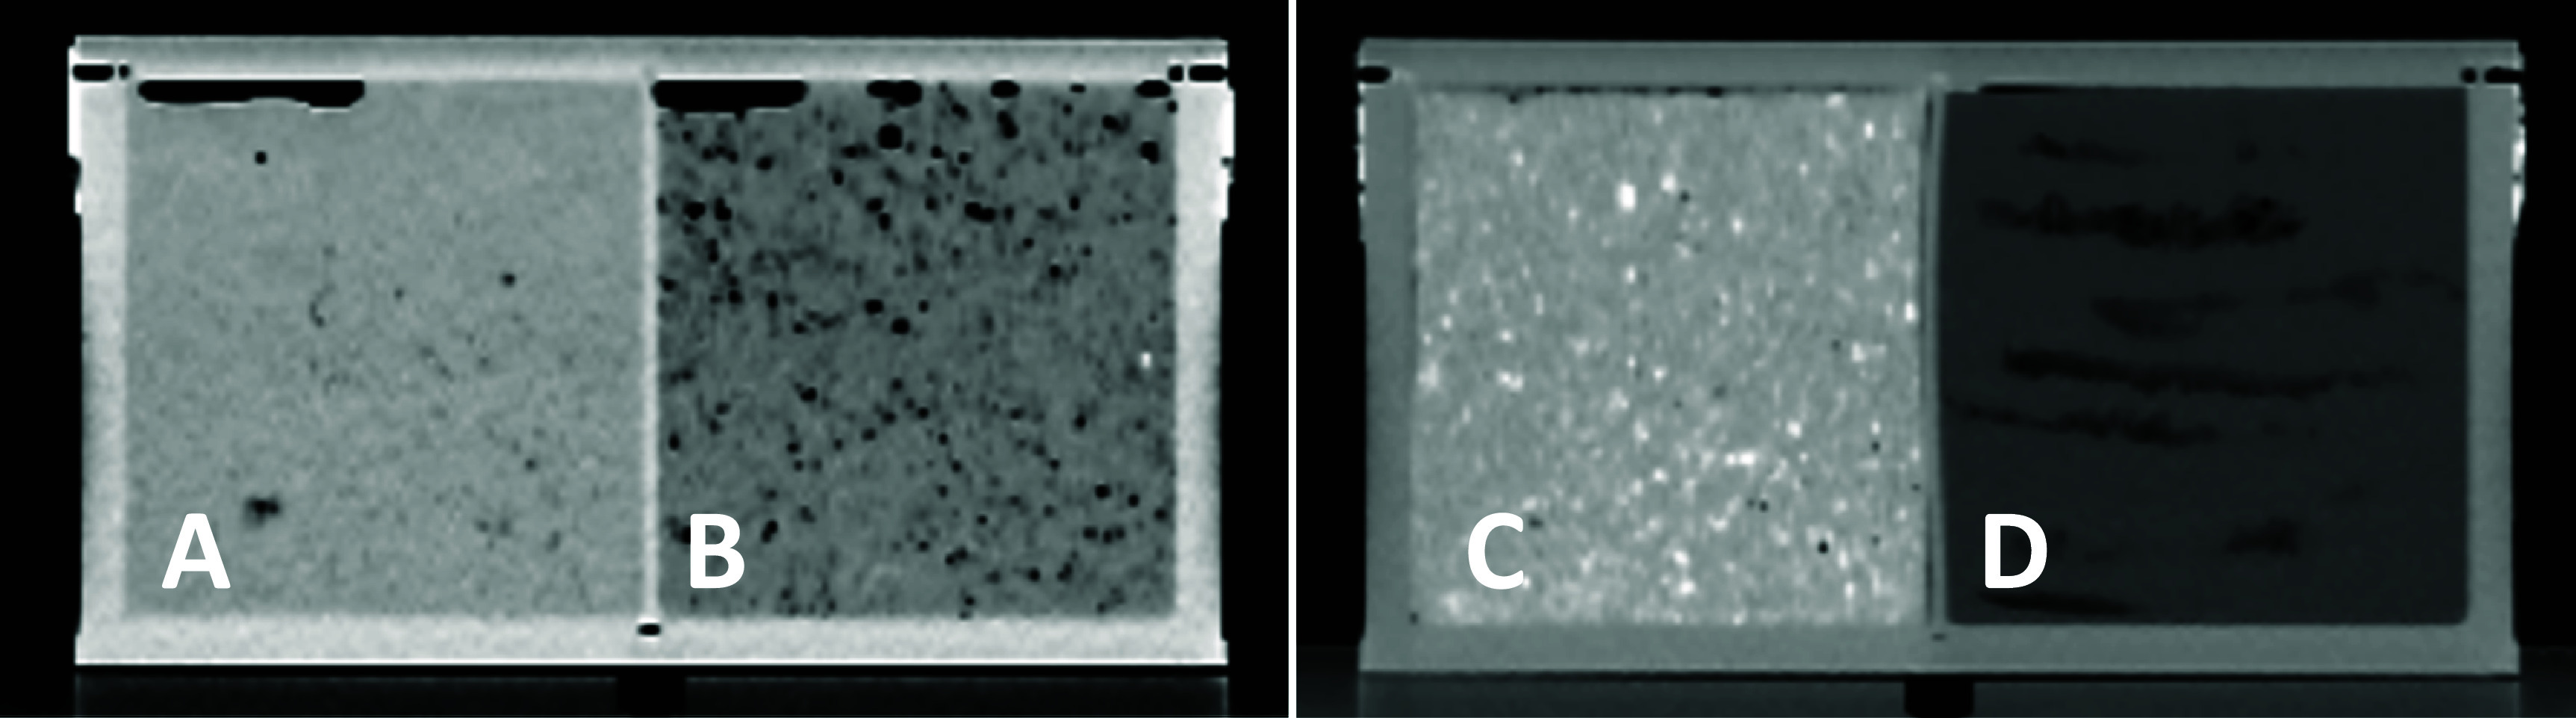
(left) Low-energy CT images of liver (A) and brain (B) (window = 400, level = 0) and (right) spongiosa- (C) and adipose-mimicking (D) samples (window = 700, level = 0). The brain sample exhibits inhomogeneities due to microbubbles of air.
